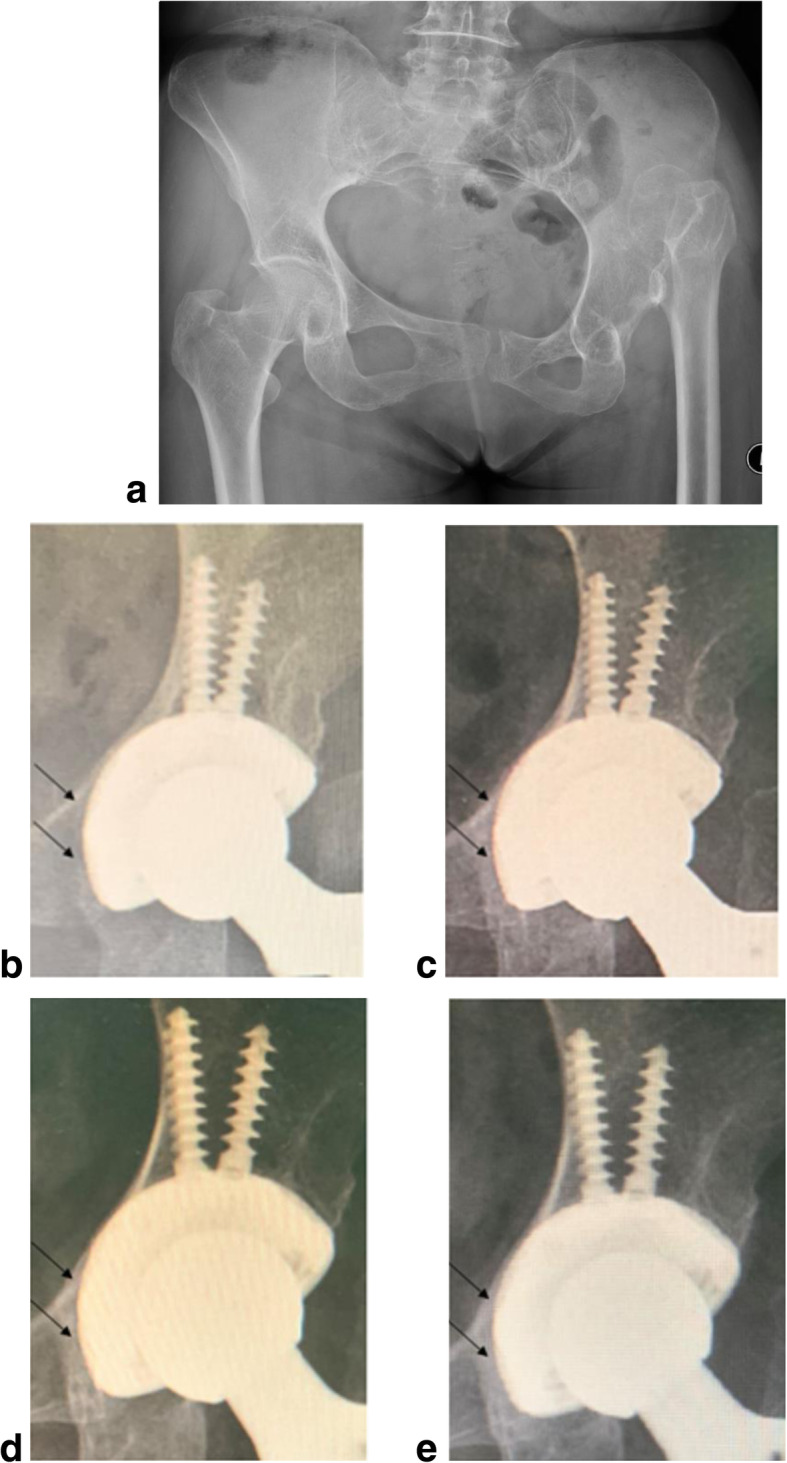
The picture shows the radiographic assessments of a 61-year-old female patient with Crowe IV DDH, preoperatively (a) and 2 weeks (b), 3 months (c), 6 months (d), and 4 years (e) postoperatively. Radiolucent line (appeared on 4b and 4c, black arrow) vanished at about 6 months postoperatively represents new bone ingrowth
